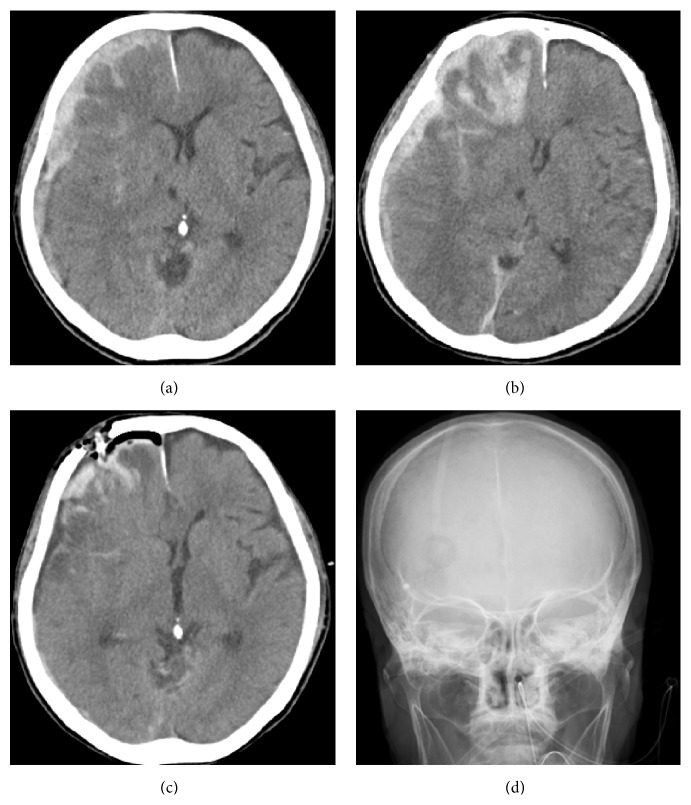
(a) Axial plain CT scan at the time of admission shows right acute subdural hematoma and right frontal and temporal contusion with thickness of 10 mm and midline shift of 6 mm. There is a bruised area in the left temporal region without bone fracture. (b) Axial plain CT scan 48 hours after admission showed massive contusion and uncal herniation. The midline shift has worsened to 9 mm. (c) Most hematoma was removed and midline shift was completely resolved. The massive contusion in the right frontal lobe was reduced. The information drain was inserted into the subdural space. (d) Radiographic frontal view shows location of the burr hole 3 cm above the right eyebrow.
